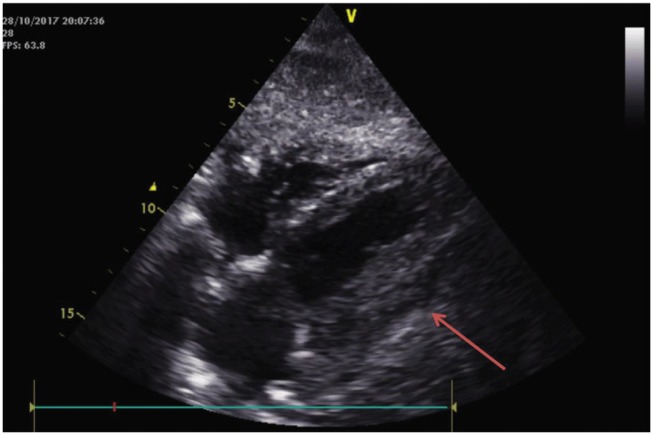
Transthoracic echocardiography four-chamber subcostal view showing a echogenic mass adjacent to the lateral left ventricular wall.
